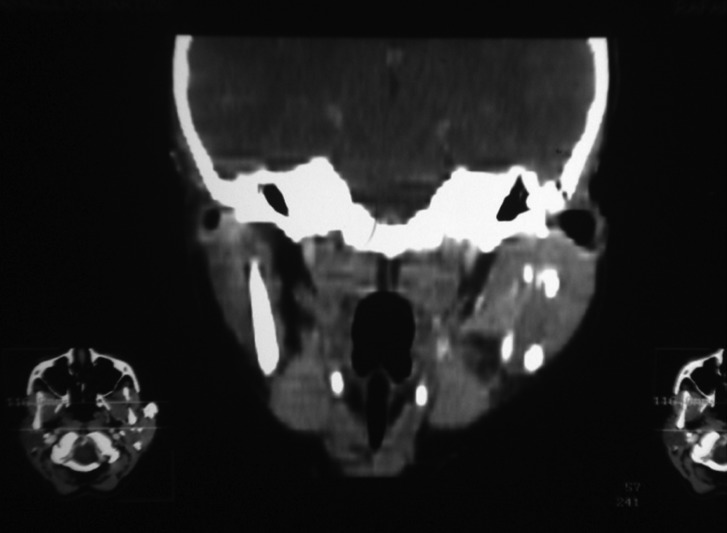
CT Scan – coronal view - dense mass in the left parotid, showing heterogeneous aspect and calcifications in its center.
